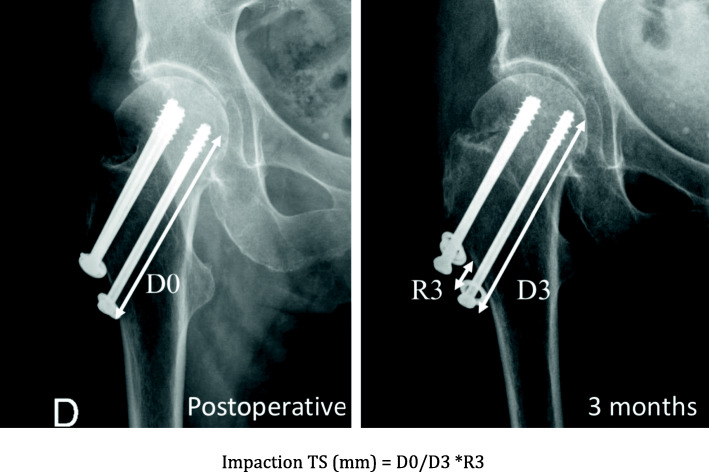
Calculation of impaction with TS implant. Postoperative and 3-month X-ray
